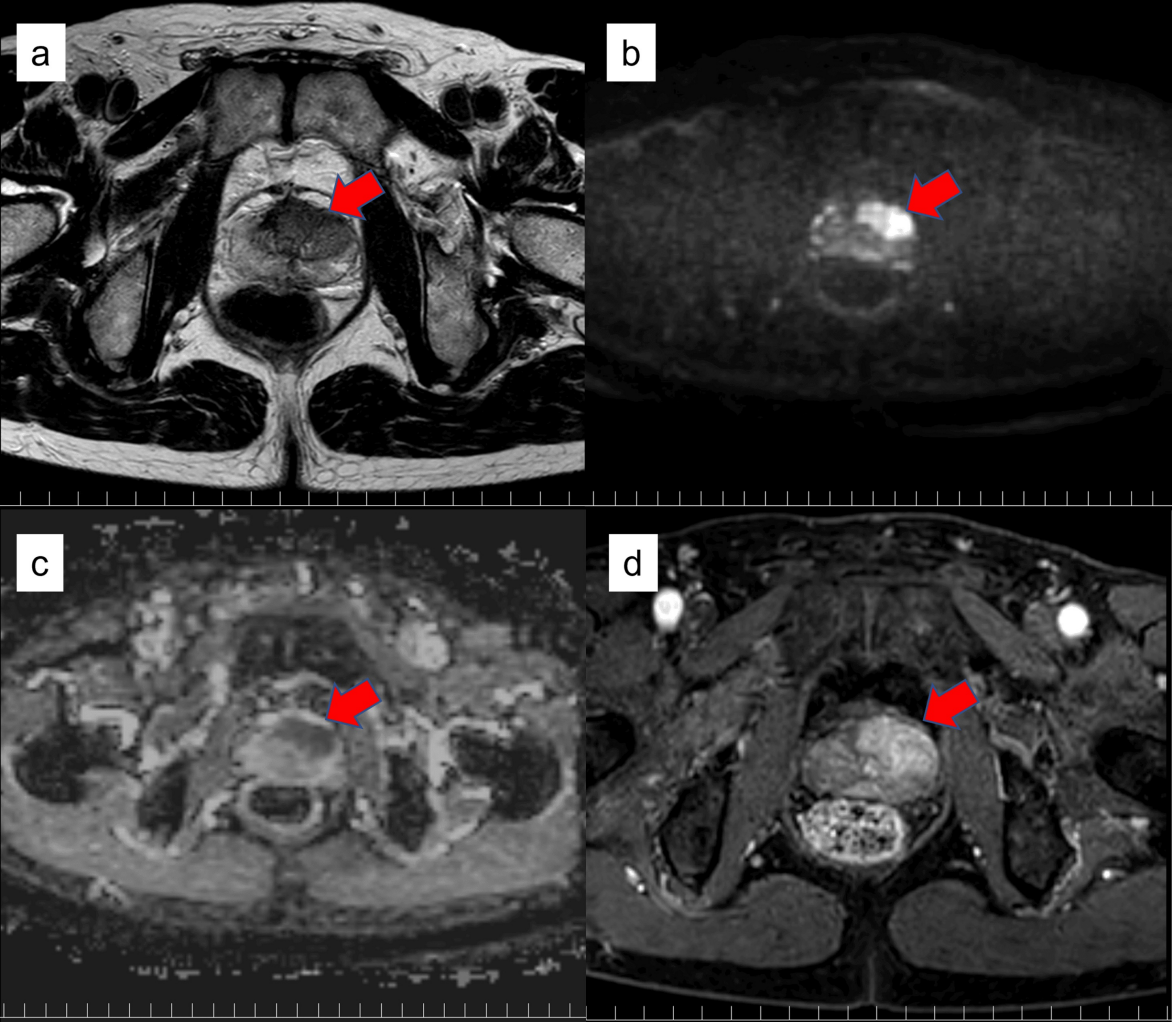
Preoperative multiparametric magnetic resonance imaging of the prostate An extensive lesion suspicious for malignancy, indicated by red arrows, was identified in the ventral aspect of the left prostatic lobe (a: T2-weighted image; b: diffusion-weighted image; c: apparent diffusion coefficient map; and d: contrast-enhanced image).
